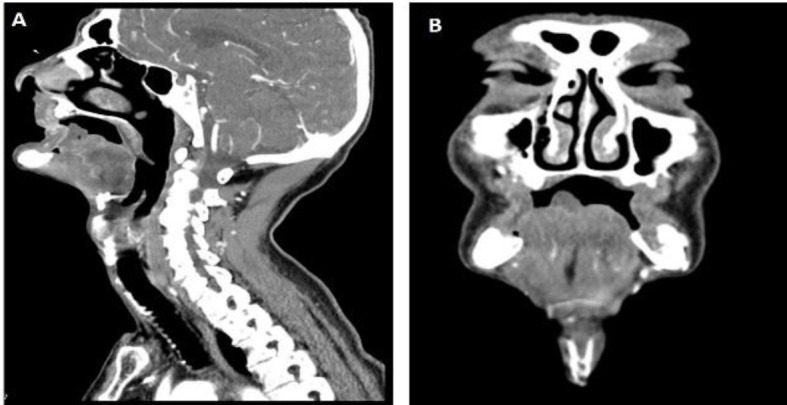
Contrast enhanced CT of the head and neck, sagittal (A) and coronal (B) views
